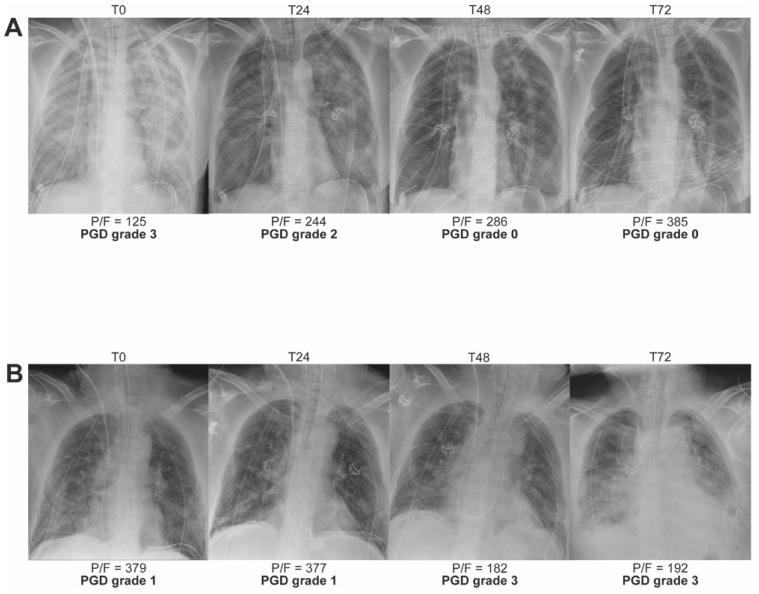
Two phenotypes of PGD grade 3 after LTx with serial chest X-rays (CXR) from two LTx recipients taken at time-point T0/24/48/72 after reperfusion of the second lung. (A) CXRs visualizing the phenotype with early transient PGD grade 3. The patient underwent sequential single-LTx via bilateral anterior thoracotomy for end-stage emphysema without extracorporeal life support (ECLS). (B) CXRs visualizing the phenotype with increasing and late PGD grade 3. The patient underwent sequential single-LTx via bilateral anterior thoracotomy for end-stage emphysema without ECLS. P/F = PaO_2_ or partial pressure of arterial oxygen/FiO_2_ or fraction of inspired oxygen.
